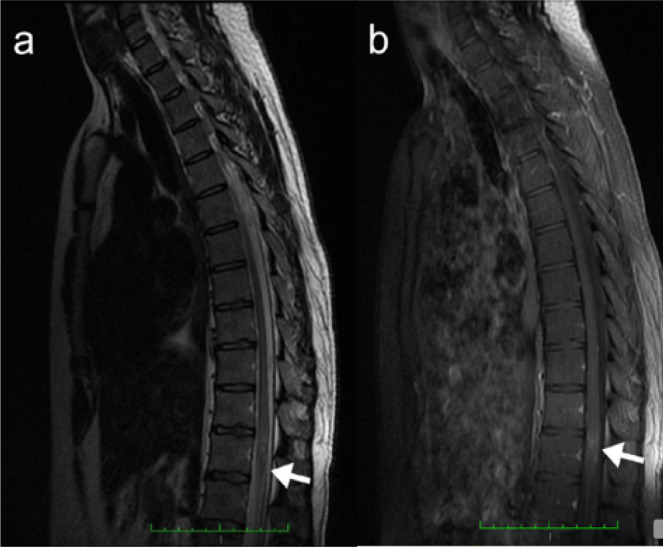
MRI scan of a patient with cerebral toxoplasmosis. Un-enhanced
